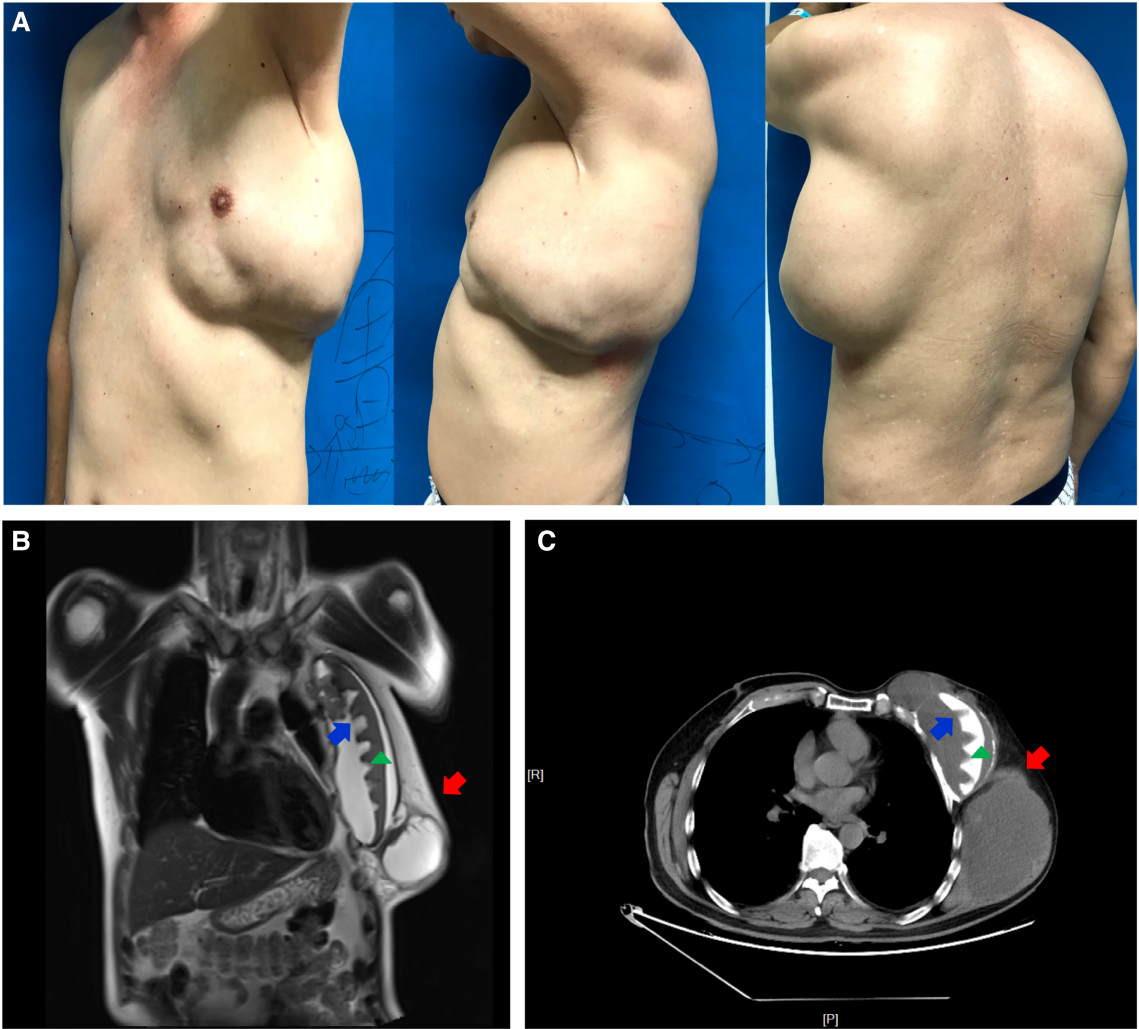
Physical examination and imaging studies of the patient. A large-sized multinodular mass was on the left chest of a 52-year-old man with a breast implant for past 19 years (A). MRI (B) and CT (C) findings showed a 20-cm sized polycystic mass (red arrow) in front of the implant (green triangle) and multiple irregular masses (blue arrow) in front of the ribs posterior to the implant.
